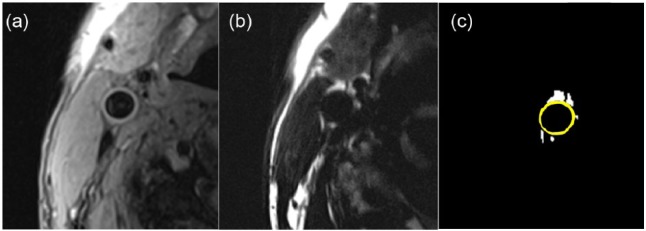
Masking effect of vessel wall and adipose tissue using Image-Pro Plus: (a) a cross-sectional T1-weighted image of a right carotid artery, (b) a matched slice of the right carotid artery using a dedicated fat saturation sequence to exhibit the PVAT and (c) the segmented PVAT using the set threshold of 2 standard deviations above the average pixel intensity values with an overlaid mask for carotid vessel wall (denoted in yellow).
